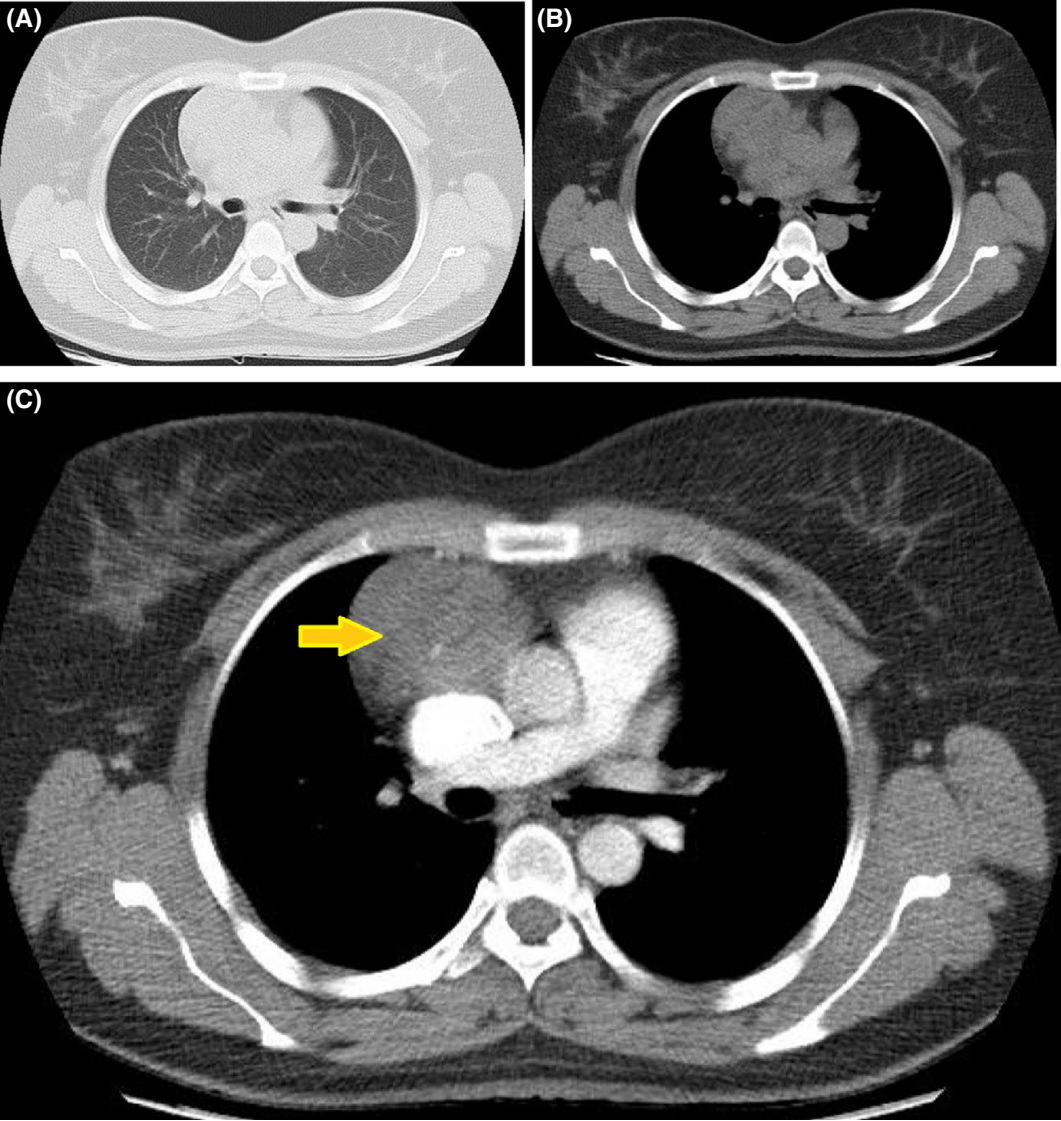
Axial CT scan demonstrating a heterogeneous lesion in the right anterior mediastinum (yellow arrow). (A): Lung window, (B): mediastinal window, and (C): after contrast administration, arterial phase
